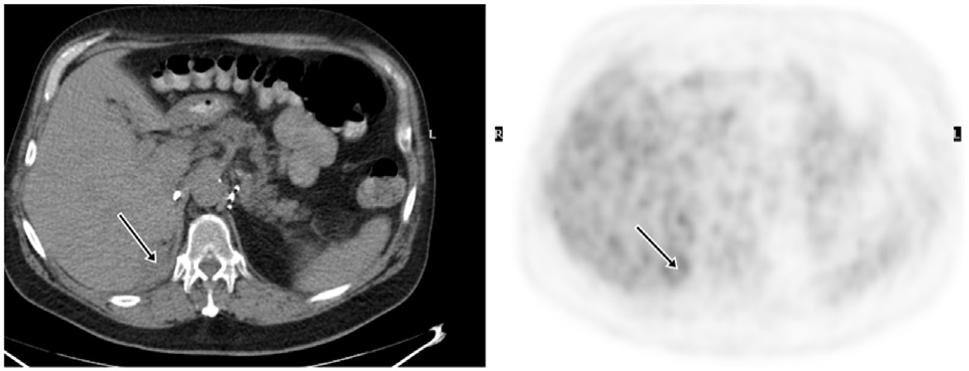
Demonstration of RCC recurrence on FDG PET/CT. A 68-year-old man had right radical nephrectomy for RCC. FDG PET/CT was obtained for surveillance 5 years later, which showed a 2.0 cm density with moderate uptake in the surgical bed and was suspicious for recurrence. Surgical pathology revealed recurrent malignancy.
